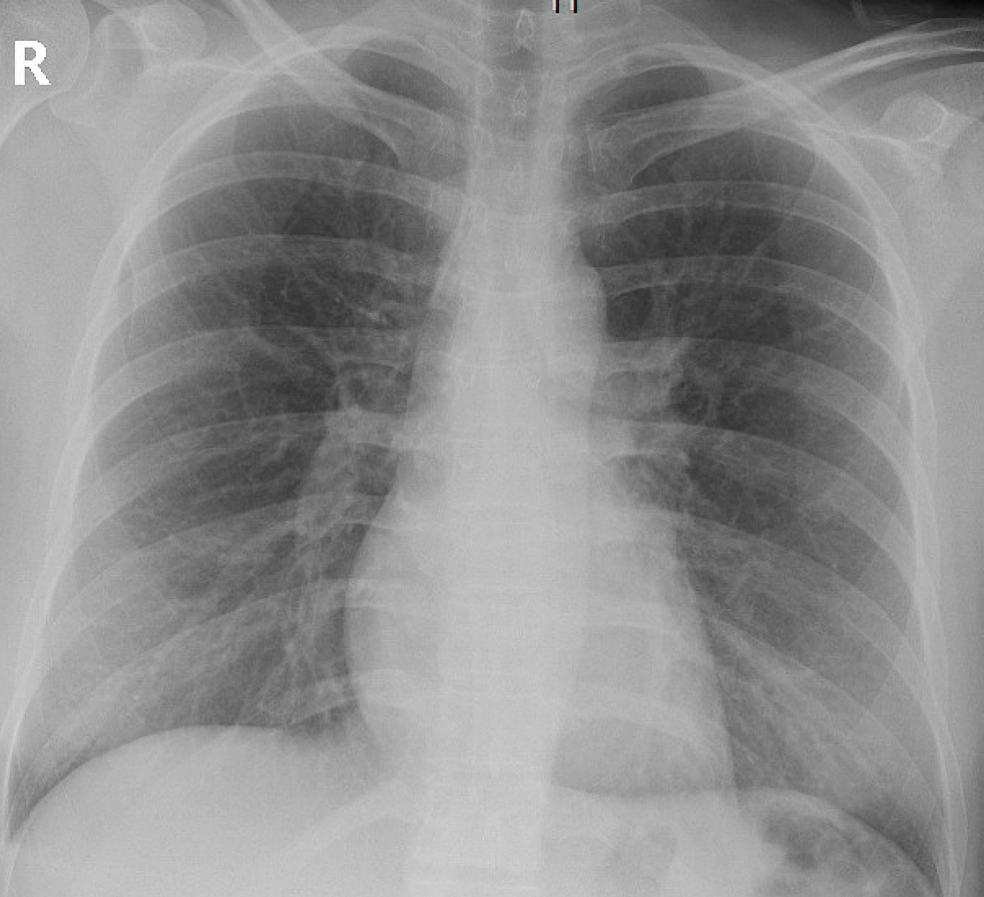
X-ray of the chest shows absence of osteolytic lesions on the ribs
